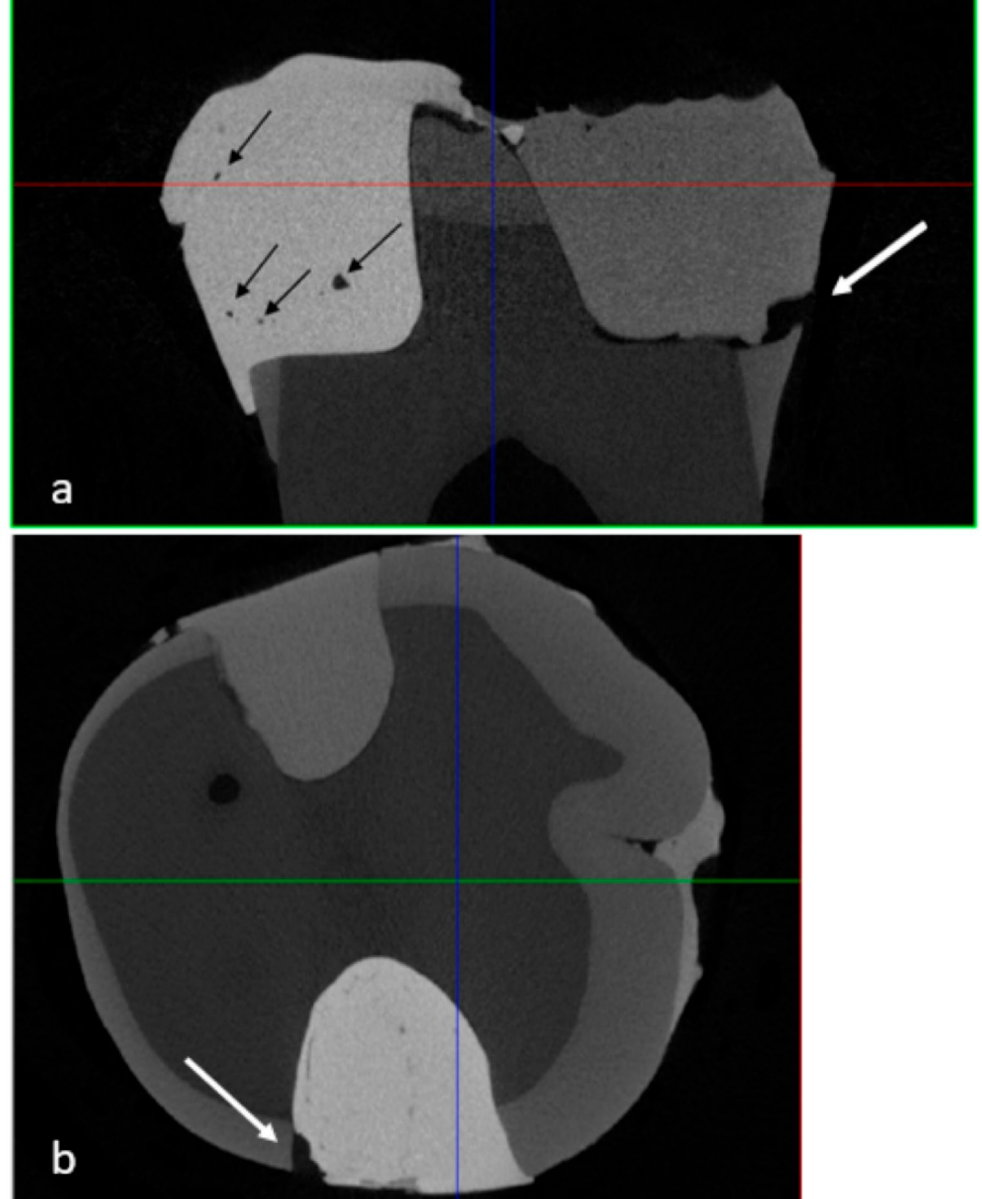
Micro-computed tomography images (a) Unacceptable gap at the gingival margin of the right-side restoration made of Filtek Supreme XTE (white arrow). Pores can also be observed inside the left-side restoration made of Admira Fusion (black arrows). (b) Unacceptable gap at the axial margin of a restoration made of Admira Fusion (white arrow)
